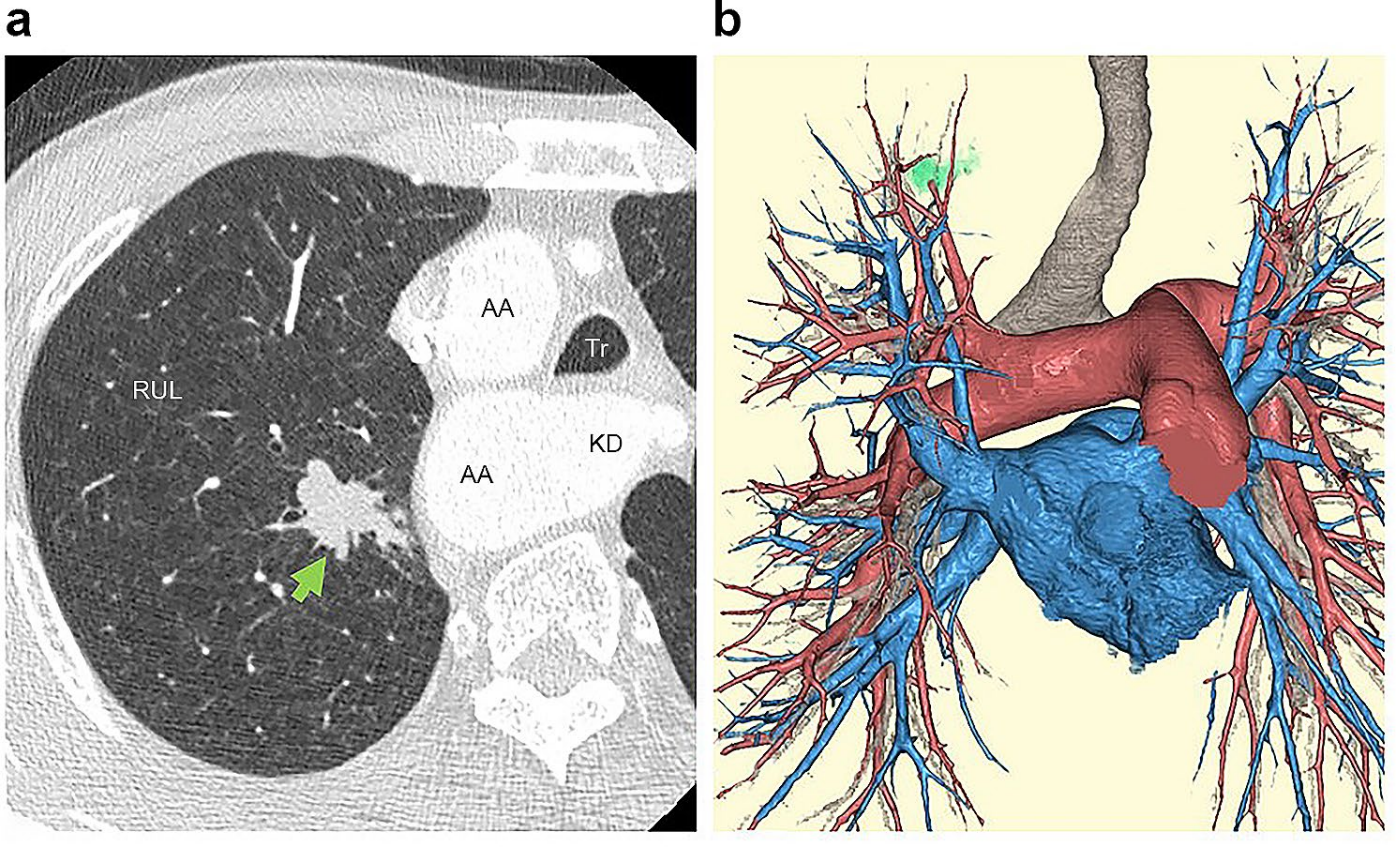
Preoperative computed tomography (CT) scan (a) The CT scan image revealed a lung nodule in the right S1 (green arrow) (b) The branching of the pulmonary artery, vein, and bronchi were normal AA, aortic arch; KD, Kommerell diverticulum; RUL, right upper lobe; Tr, trachea
